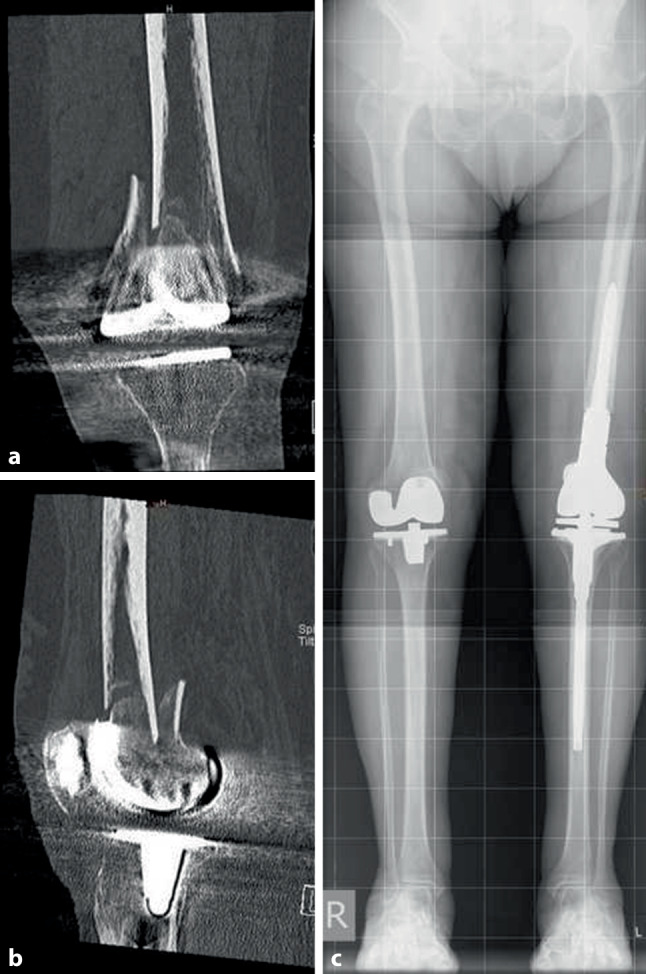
A 77-year-old female patient with PPF of the distal femur. CT scans verify type 3 fracture according to Su et al. Treatment with modular distal femur GMRS allowed for reconstruction of correct leg length and joint line
